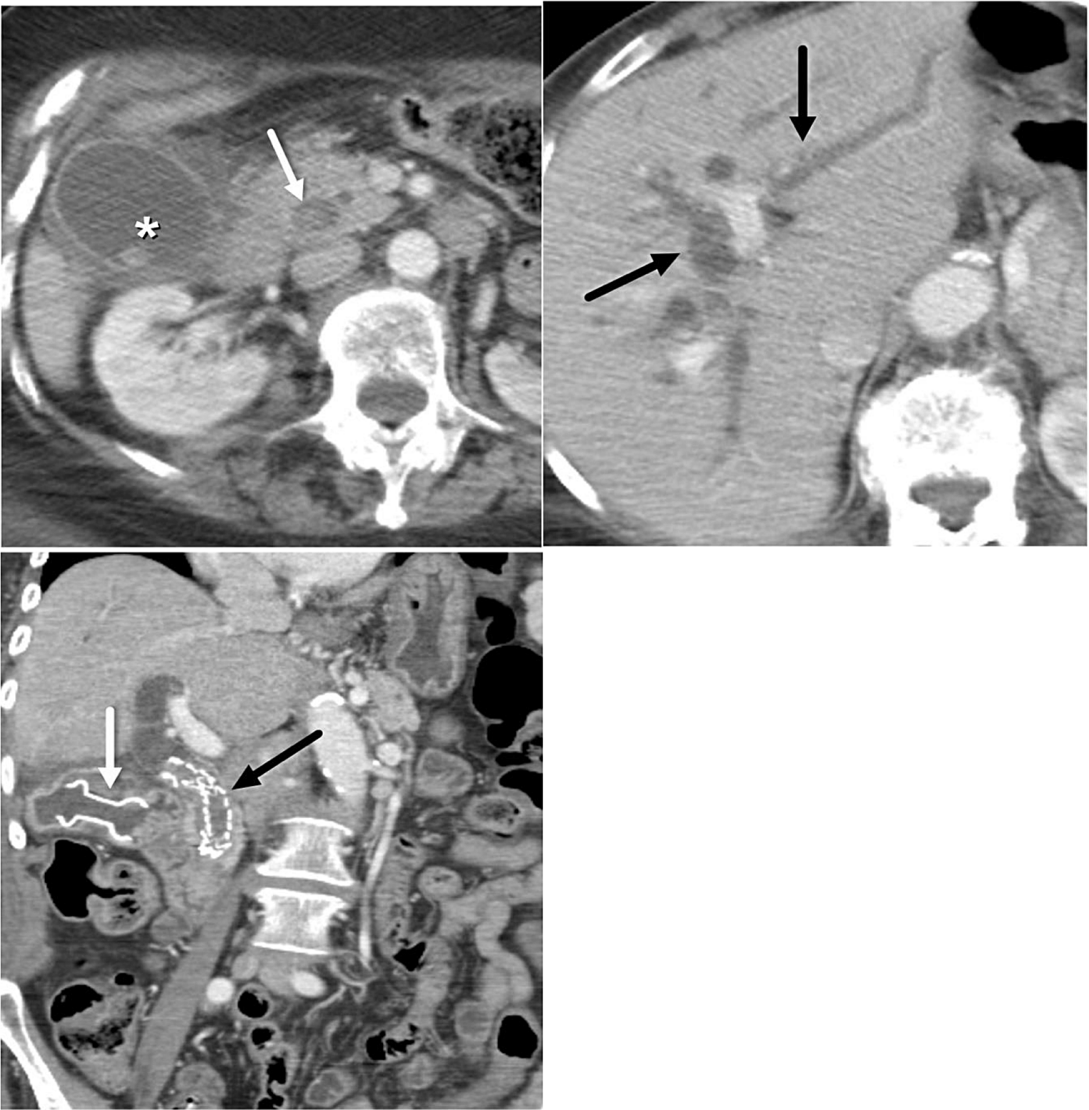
86 year-old female presented with acute cholecystitis complicated by ascending cholangitis due to choledocholithiasis. A. Contrast-enhanced axial CT image shows cholelithiasis, gallbladder wall thickening, and pericholecystic fluid of acute cholecystitis (white asterisk) complicated by ascending cholangitis due to choledocholithiasis (white arrow). B. Contrast-enhanced axial CT demonstrates moderate bilobar intrahepatic biliary dilatation (black arrows). C. Contrast-enhanced coronal CT image shows LAMS (white arrow) draining the gallbladder into the duodenum and a biliary stent (black arrow) placed during the same ERCP session in this patient who was a poor surgical candidate. Both the biliary stent and LAMS remained in place for 5 years. The patient had one subsequent bout of cholangitis and ultimately passed away at the age of 92 of unrelated causes
